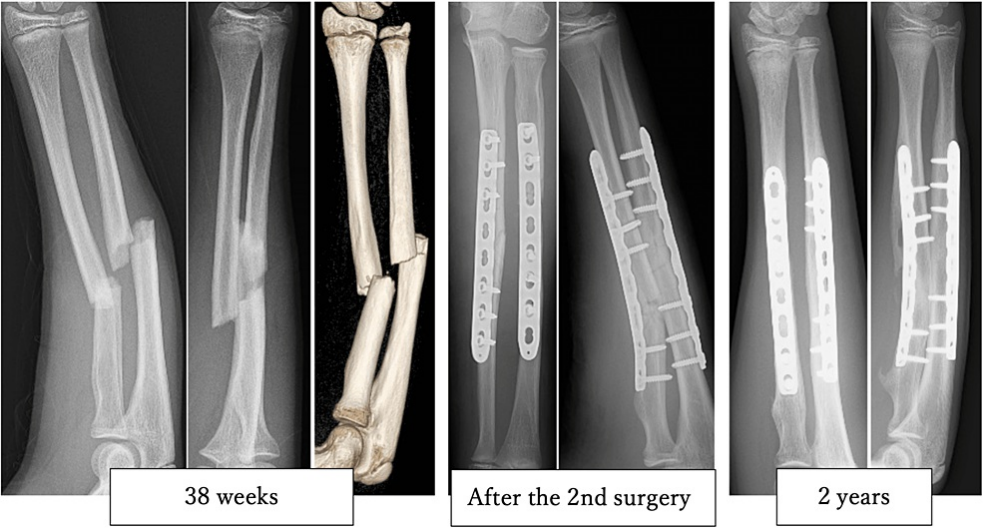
Subsequent image of the aforementioned 14-year-old male X-rays taken at 38 weeks, and at 2 years, revealed that the radius and ulna had re-fractured; the bones were then double-plated for stabilization within the same week. The bones fused properly post-operation and remained stable for 2 years afterward.
